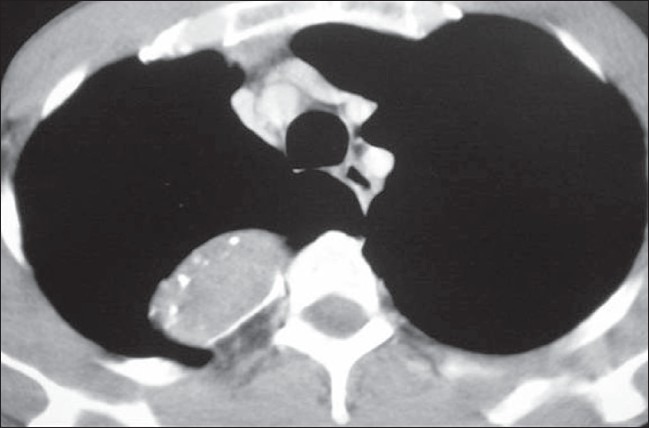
CT scan of the thorax showing a posterior mediastinal neurogenic tumour with no intraspinal extension
